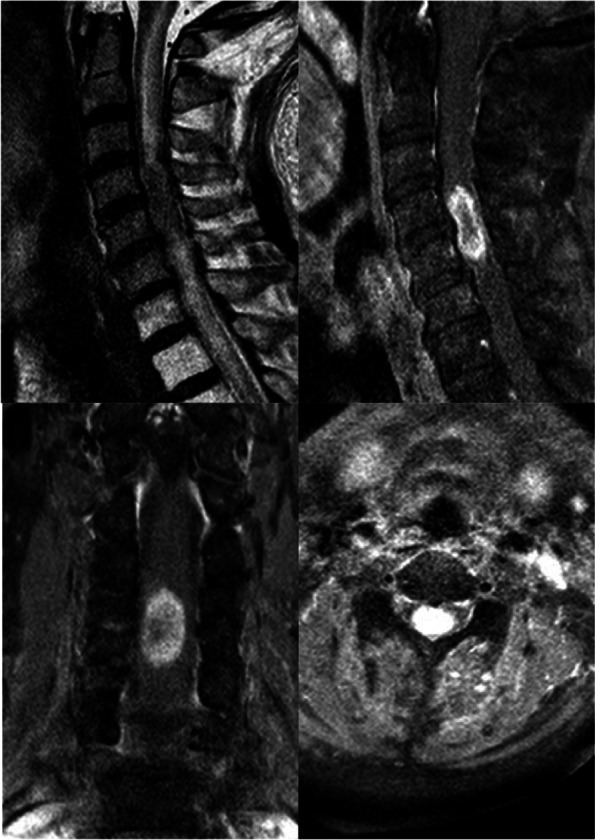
Sagittal, coronal and axial T1-weighted post-gadolinium enhanced imaging demonstrated a mixed-intense tumor with typical more intense thin rim of peripheral enhancement
